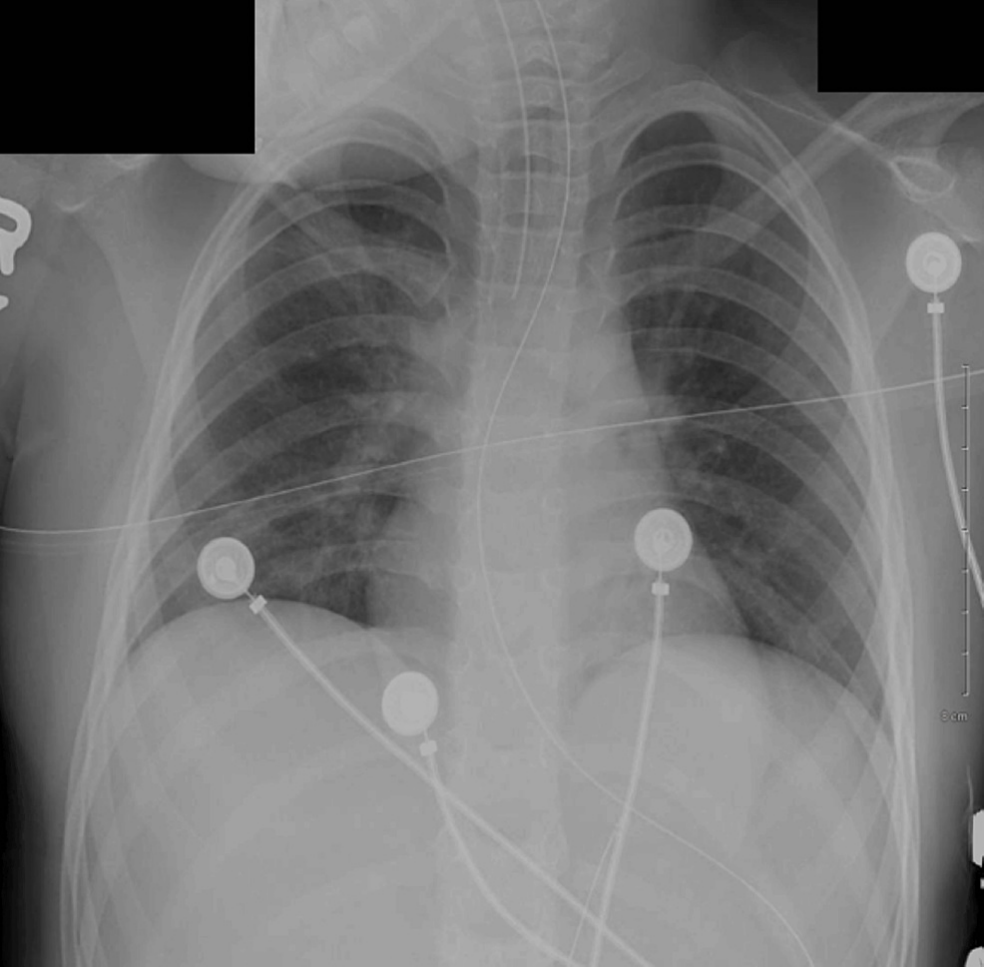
The chest X-ray taken after ET placement upon admission. ET: endotracheal tube
